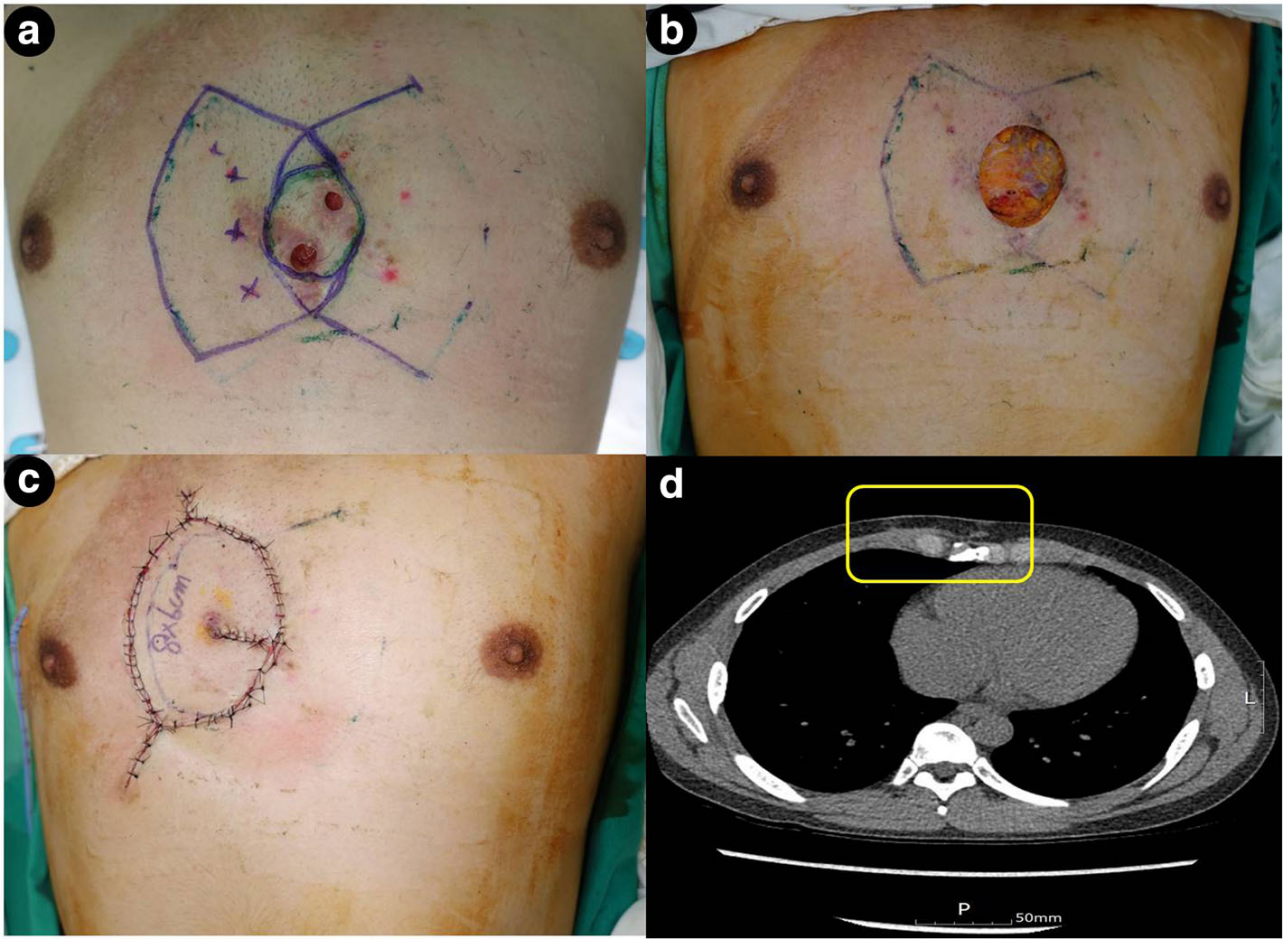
a A 29-year-old man with wound dehiscence following a keloid excision with direct primary closure on the anterior chest wall, performed at another clinic. Marking of cutaneous perforators, identified using a handheld Doppler preoperatively, and design of the keystone flap. The wound was infected with methicillin resistant *Staphylococcus aureus* (MRSA). b Intraoperative view of a defect, measuring 5.5 × 5.0 cm, after resection of an infected wound. c Immediate postoperative result of the fortune cookie design perforator island flap and d Computed tomography scan in axial plane at 2 months’ follow-up
